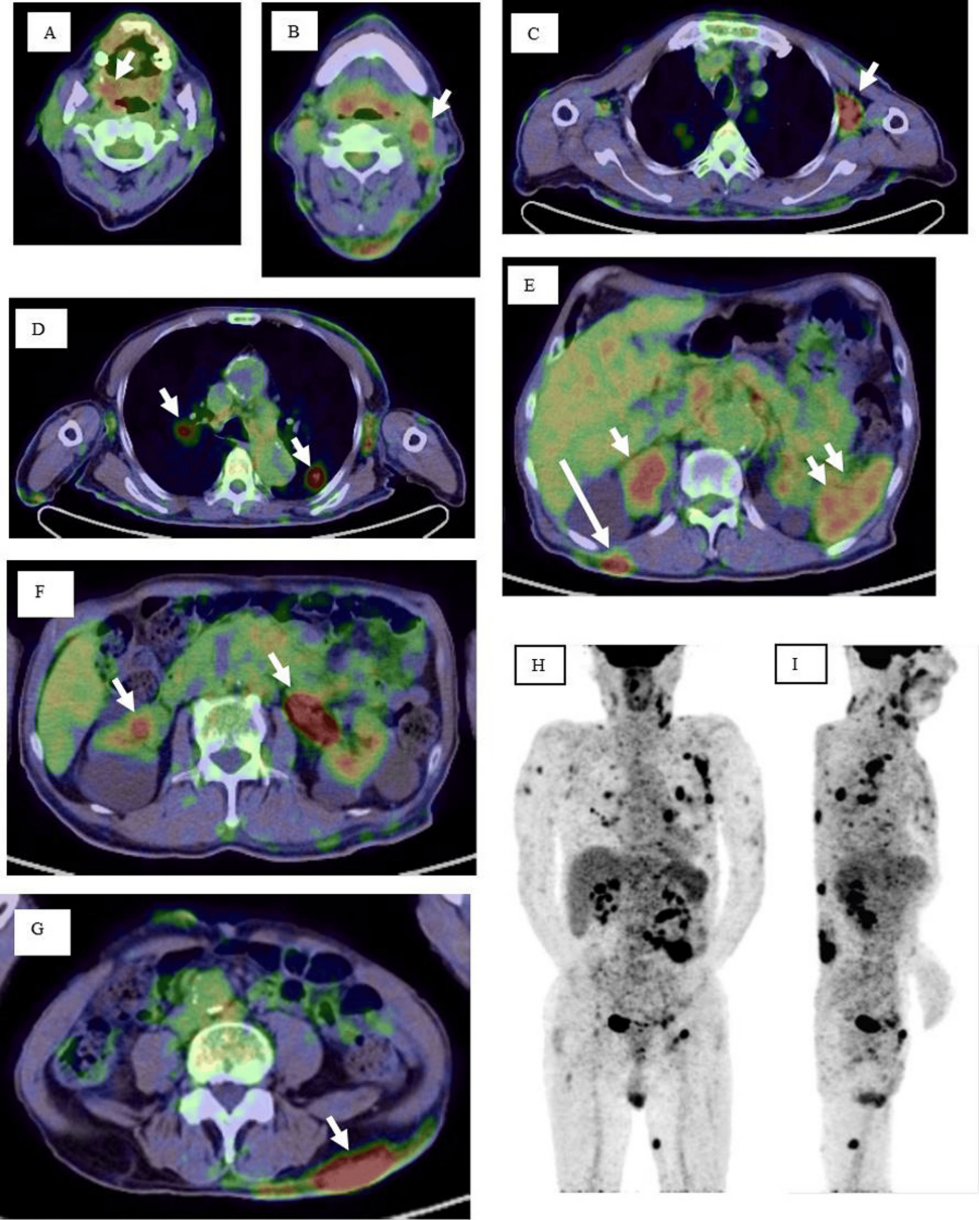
PET-CT performed in April in the third year of onset The abnormal uptake of ^18^F-fluorodeoxyglucose (FDG) is indicated by a red hue and pointed out by arrows. A: pharynx, B: lymph nodes of the neck, C: axilla, D: lung field, E and F: abdominal lymph nodes. In E, double arrows indicate the uptake in the spleen, and a long arrow indicates the surface of the back skin. G: back skin. H, I: maximum intensity projection in the early phase. Frontal view (H) and side view (I). The FDG uptake is shown in black. FDG accumulation in the brain and liver appears to be physiological PET-CT: positron emission tomography-computed tomography
